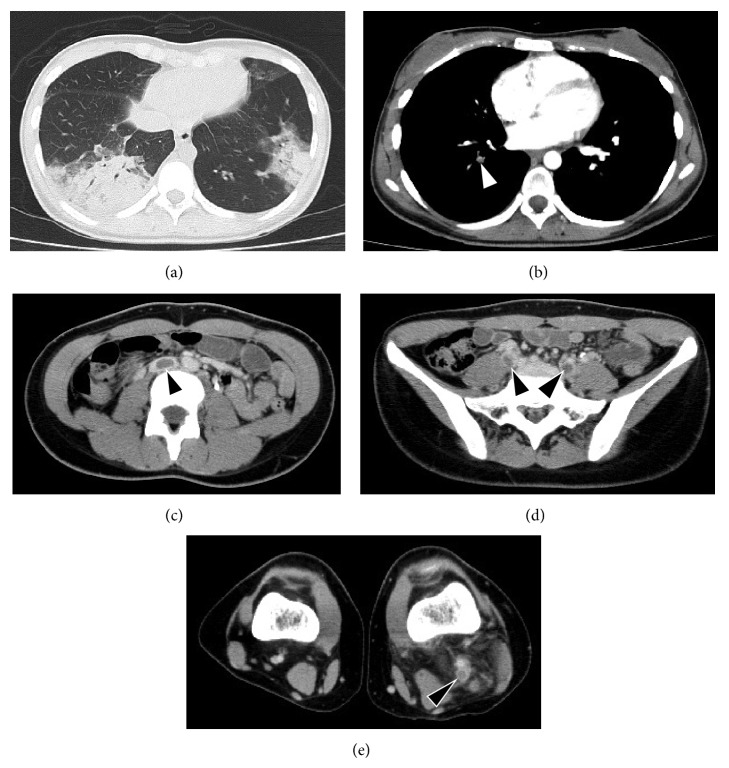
Computed tomographic scan showing consolidations in bilateral lower lungs (a), pulmonary embolism in the right pulmonary artery ((b), white arrowhead), and extensive DVT throughout inferior vena cava to left posterior tibial vein ((c)–(e), black arrowheads).
